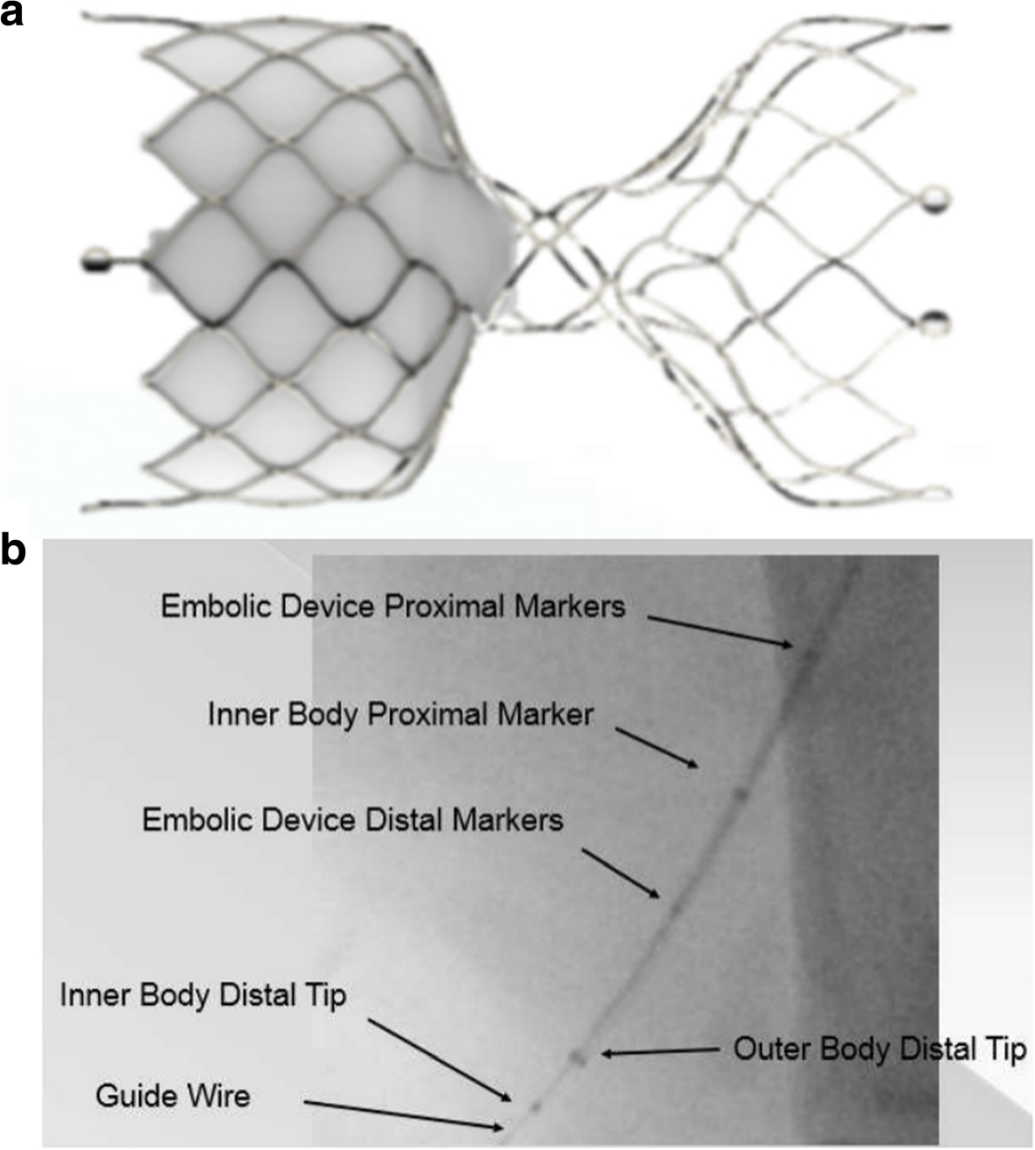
The EMBA Hourglass device: a A Nitinol frame, the proximal half of which is covered with PTFE. Unconstrained, the device measures 20 mm in length and 10 mm in diameter. b Single radiographic image showing the device and delivery system in situ with the location of the radio-opaque markers indicated
